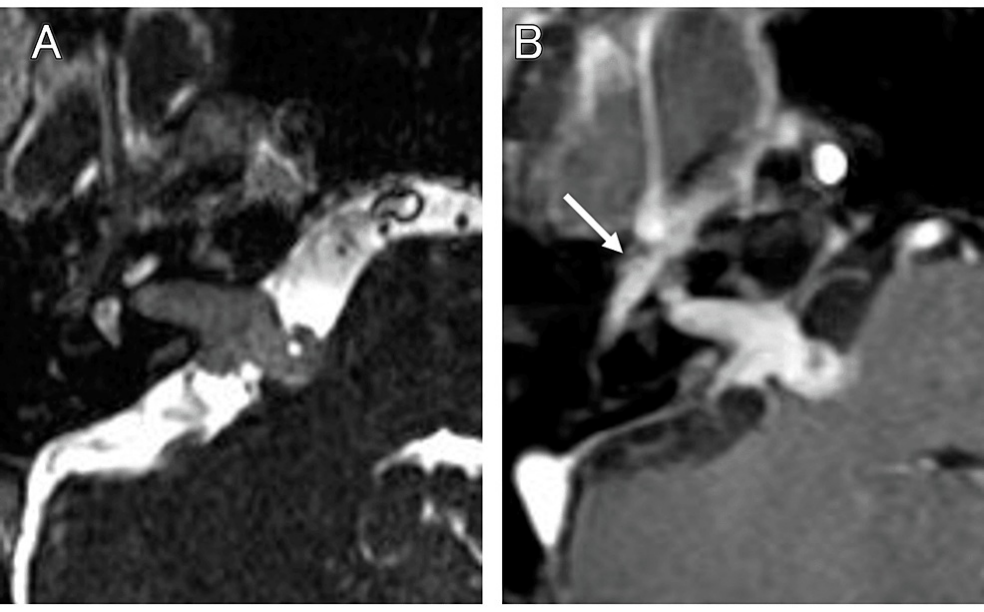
MRI three months after the initial diagnosis T2-weighted (A) and contrast-enhanced T1-weighted (B) images show that the tumor has grown and is in contact with the brainstem. The tumor in the internal auditory canal extends into the geniculate ganglion segment (arrow). MRI: magnetic resonance imaging
